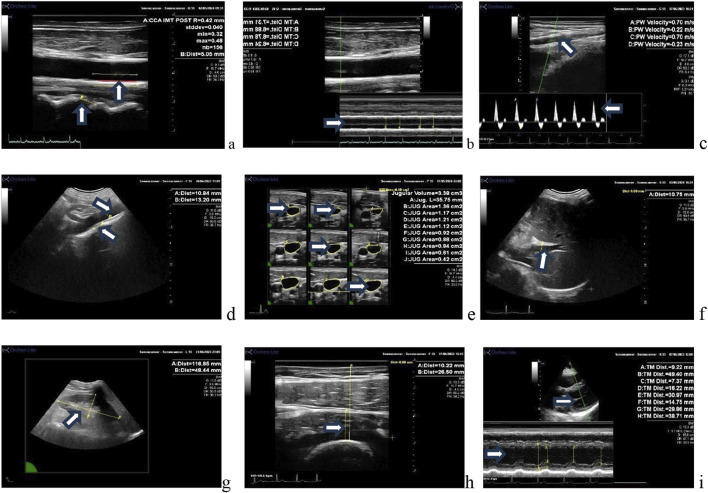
Ultrasound images of various vessels/organs assessed in this study. (A) Longitudinal view of the common carotid artery intima media thickness and cervical intervertebral distance, (B) carotid anterior and posterior wall time motion trace (systolic and diastolic diameter), (C) tibial artery image and Doppler velocity (lower limb vascular resistance). (D) Abdominal aorta diameter and lumbar intervertebral distance; (E) jugular vein volume (3D display); (F) portal vein diameter, (G) right kidney long axis area; (H) vastus intermedius thigh muscle thickness; (I) left ventricle parasternal long axis (volume, stroke volume posterior wall thickness).
